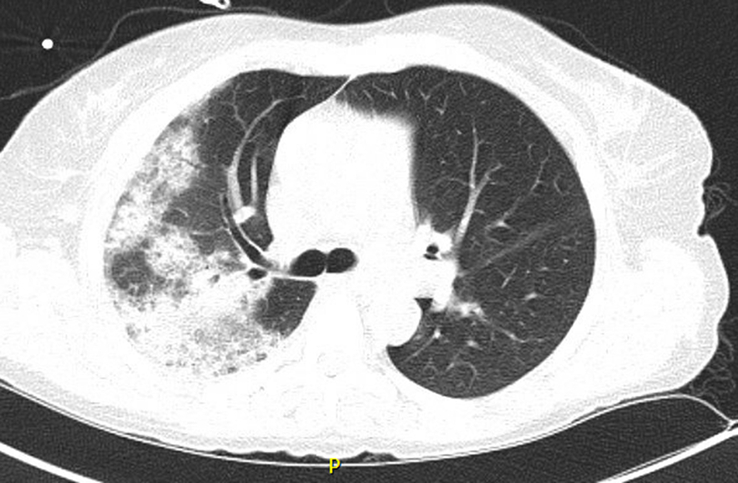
Computed tomography chest shows airspace consolidations involving the right upper lobe, apical and posterior segments, and the superior segment of the right lower lobe.
